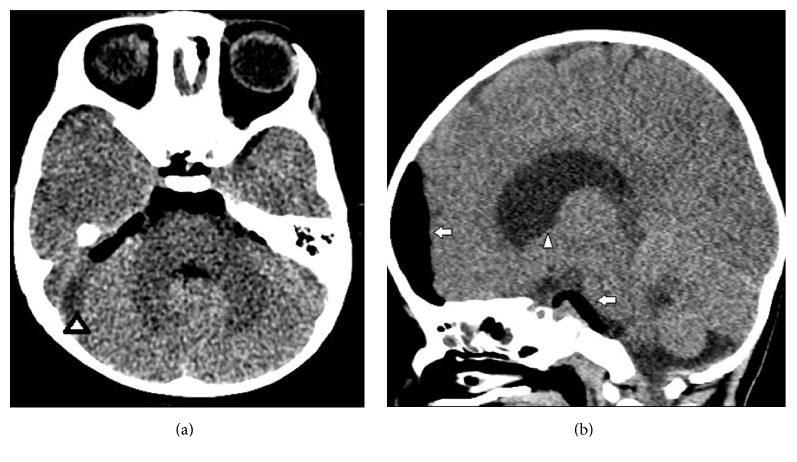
Head CT on day 2 for evaluation of meningitis. (a) Axial head CT shows subdural effusion over the right cerebellar hemisphere abutting the sigmoid sinus (arrowhead) and large air in the prepontine cistern. (b) Sagittal reformat demonstrates large pneumocephalus over the left frontal lobe and in the prepontine cistern (arrows). There is mild lateral ventricle dilatation (arrowhead).
